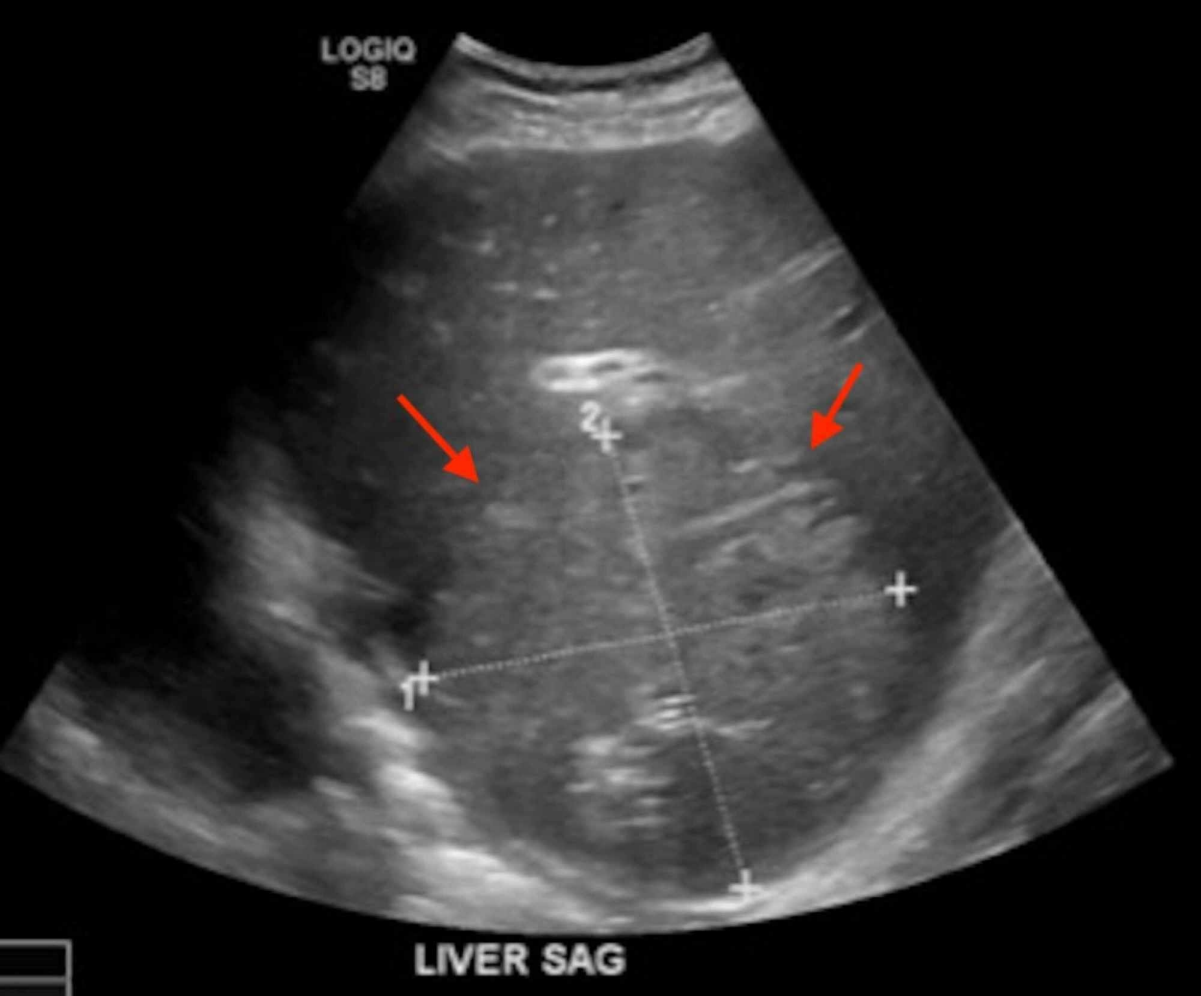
Ultrasonography of the abdomen demonstrating an increase in the size of the abscess to 12.3 x 11.9 cm after hepatic drain placement. The arrows point to area of interest.
